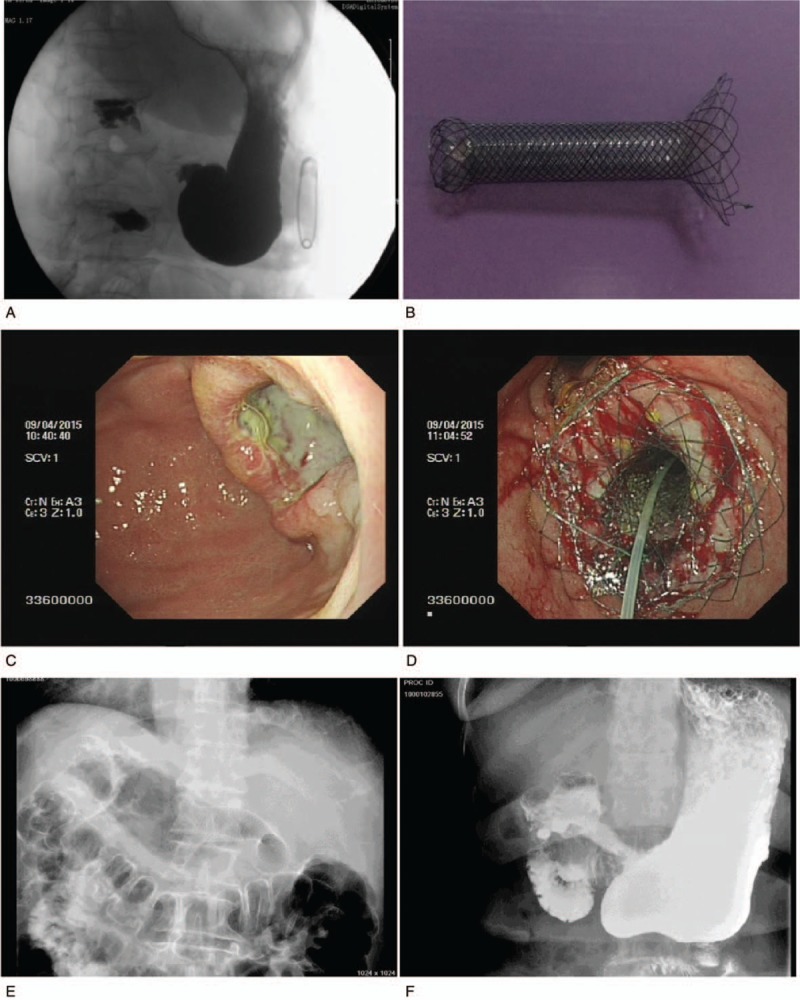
Use of a partially covered funnel stent in treatment of cup shaped GOO. A, Distal antrum cup-shaped obstruction. B, Model of funnel stent used to resolve the obstruction. C, Endoscopic view of a tumor in the gastric antrum. D, Endoscopic view of the proximal funnel stent at the pyloric area. E, Confirmation of stent deployment by fluoroscopy. D, Barium contrast radiography shows contrast agent filling the stent. GOO = gastric outlet obstruction.
